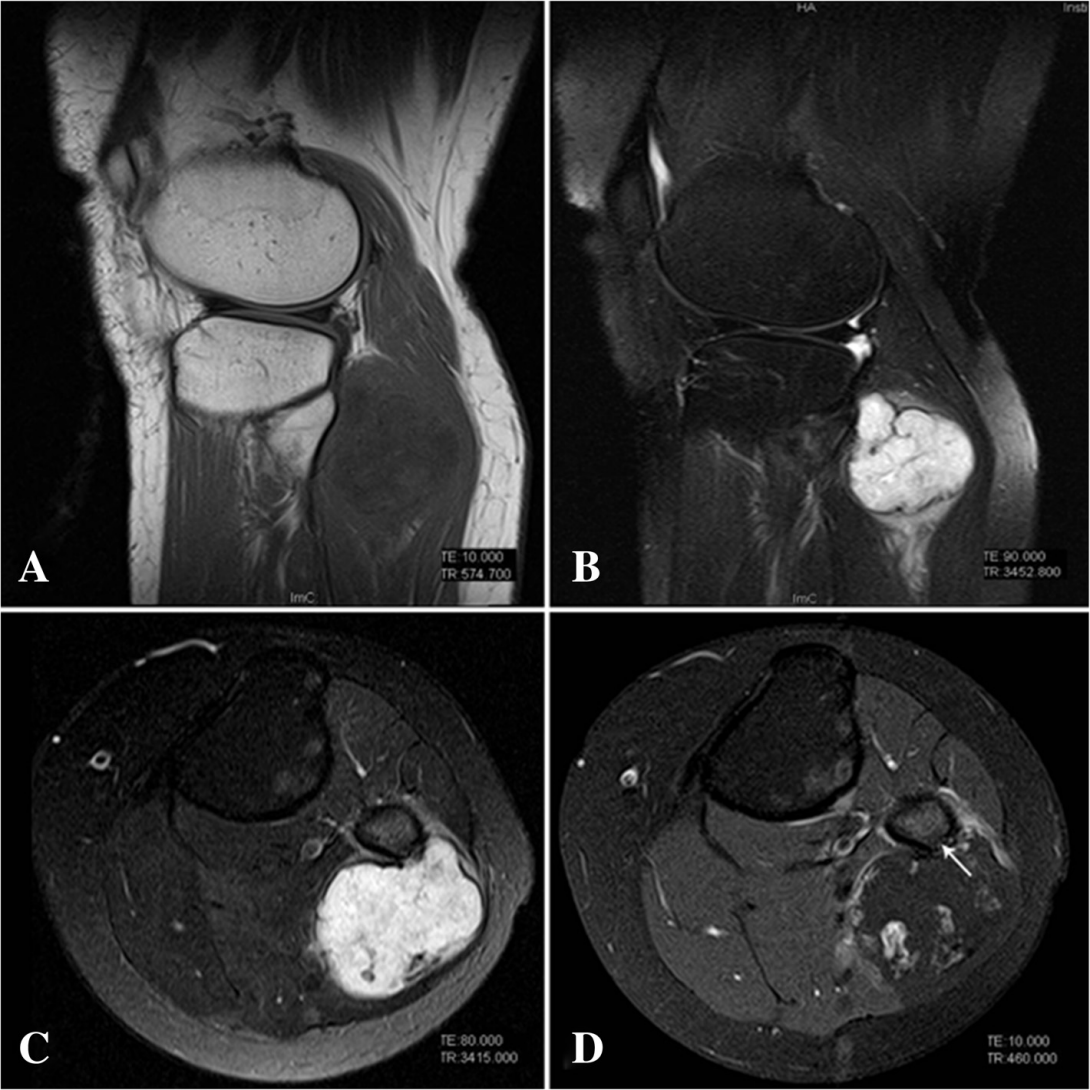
MRI of tumor. A Sagittal T1-weight image shows a homogeneous hypo-intensity mass on the posterolateral aspect of the left proximal fibula. B, C Sagittal and axial T2-weight images show a hyper-intensity mass (5.5 × 5.0 × 4.5 cm) with a multilobular configuration. D Axial T1-weighted MRI with fat suppression shows the contrast enhancement around the tumor and cortical invasion (arrow).
